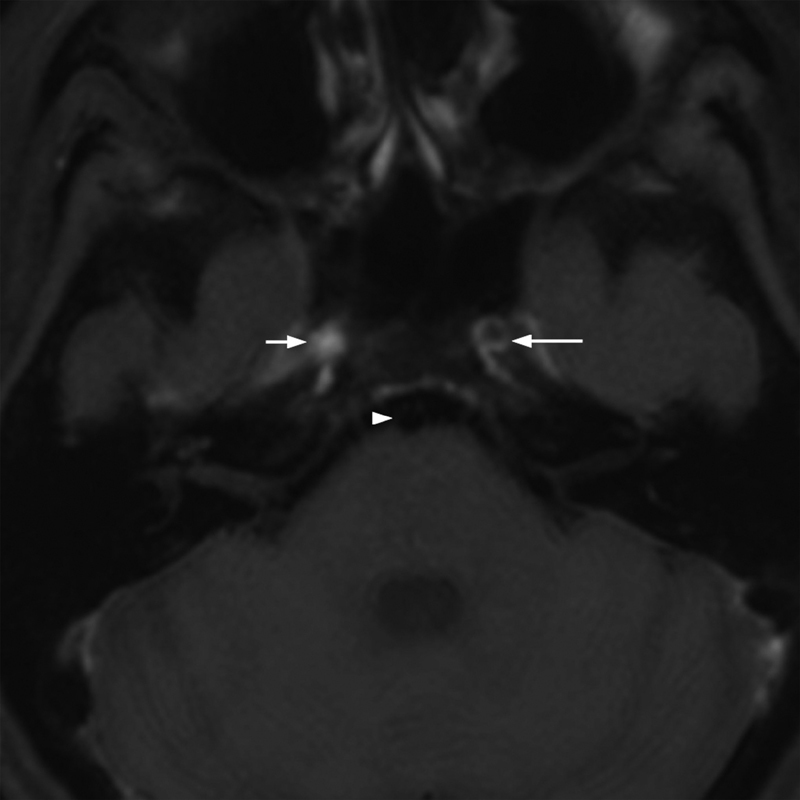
T1 black-blood post-gadolinium imaging sequence MRI showing normal basilar artery (
